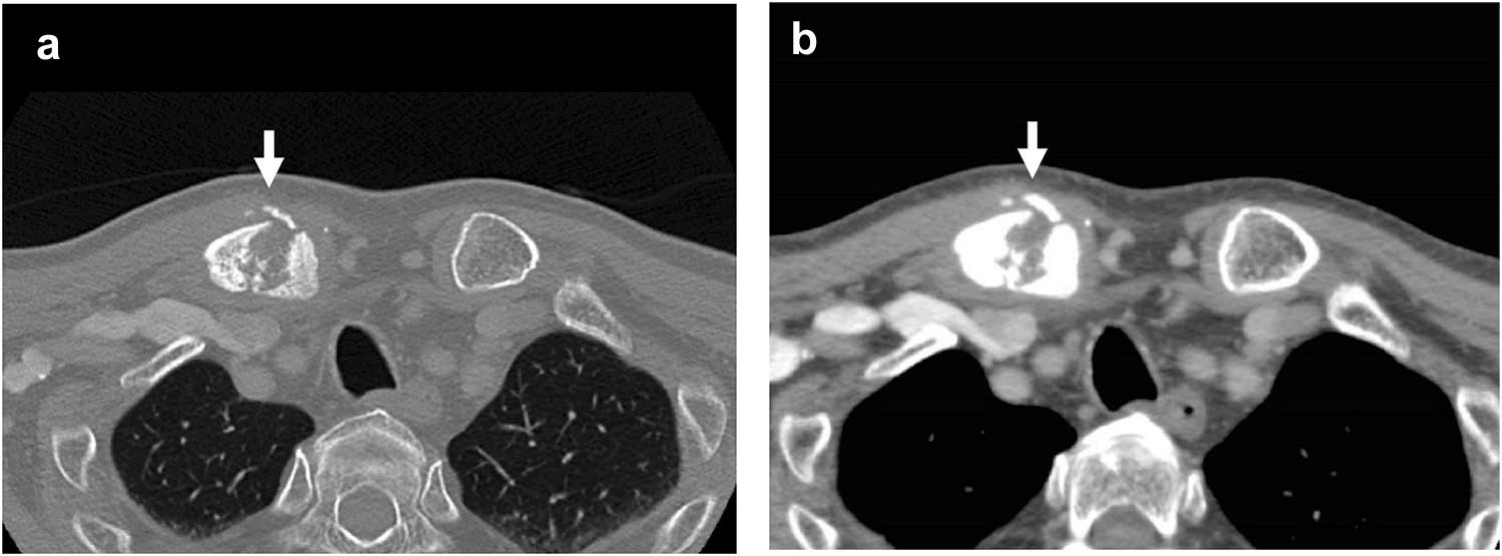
Contrast-enhanced CT (a: bone-window image, b: soft tissue-window image) on September 17, 2010, 6 months after right RND, showed a fracture with bone destruction (arrows) at the proximal end of the right clavicle
